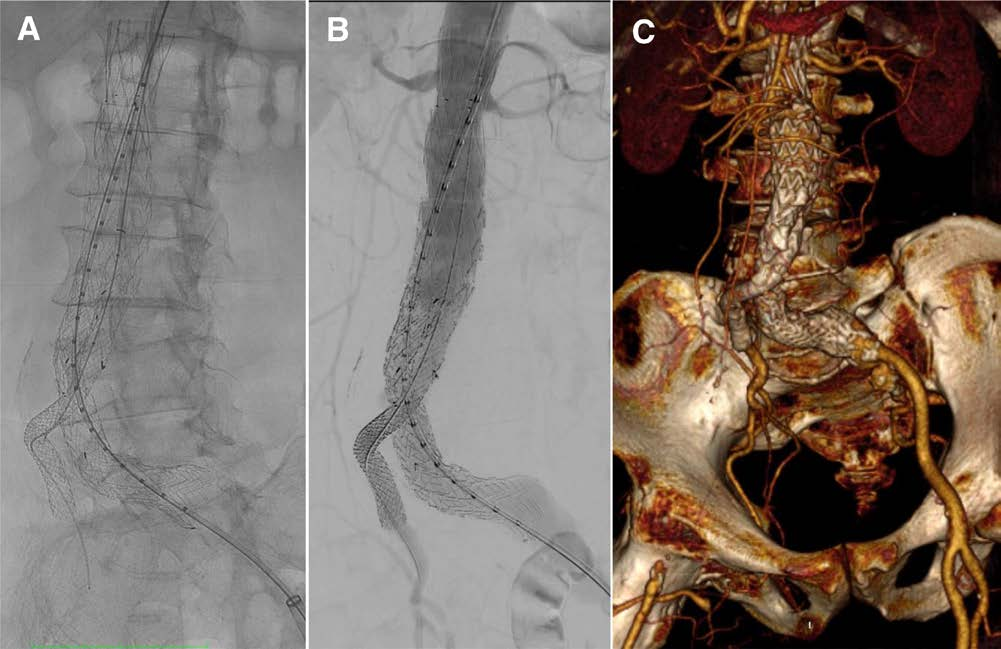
Angiographic image after stent graft placement (A). No endoleak was observed on the final intraoperative angiography (B), and postoperative CT (C). CT = computed tomography.
